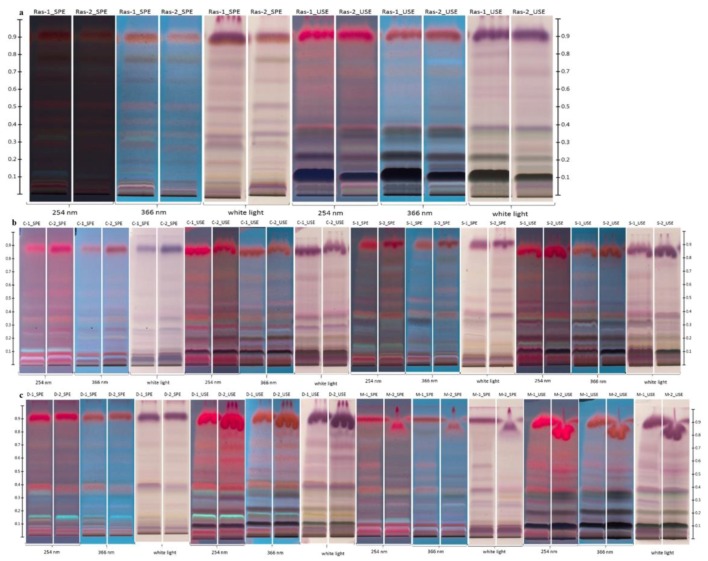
HPTLC fingerprints of honeys of different botanical origin after derivatisation (UAE—ultrasound assisted extraction, SPE—solid phase extraction visualised under white light, 254 nm and 366 nm; (a) Ras—raspberry honeys; (b) C—clover honeys; S—sweet yellow clover honeys; (c) D—dandelion honeys; M—milk thistle honeys).
